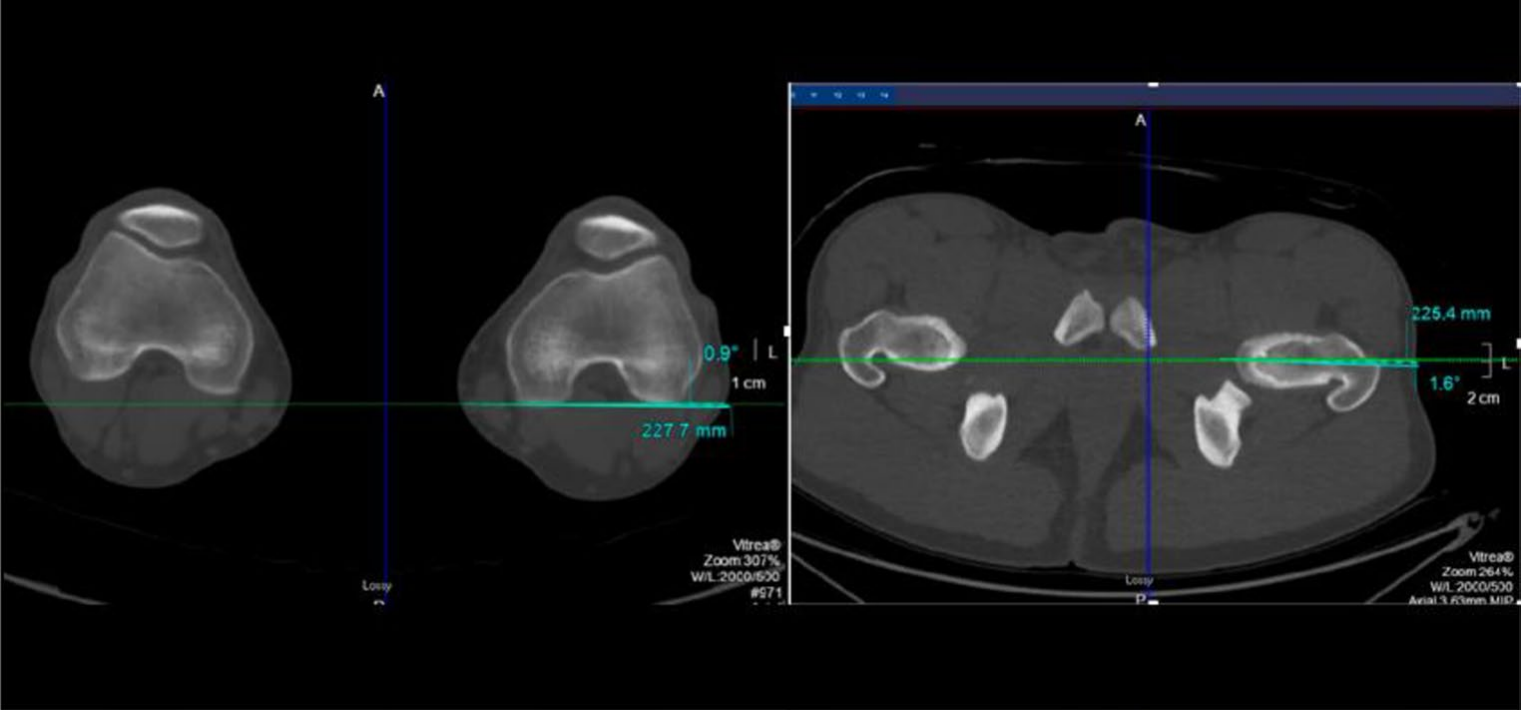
Measurement of the femoral version from the oblique axial femoral neck and axial distal femur slices of the CT
